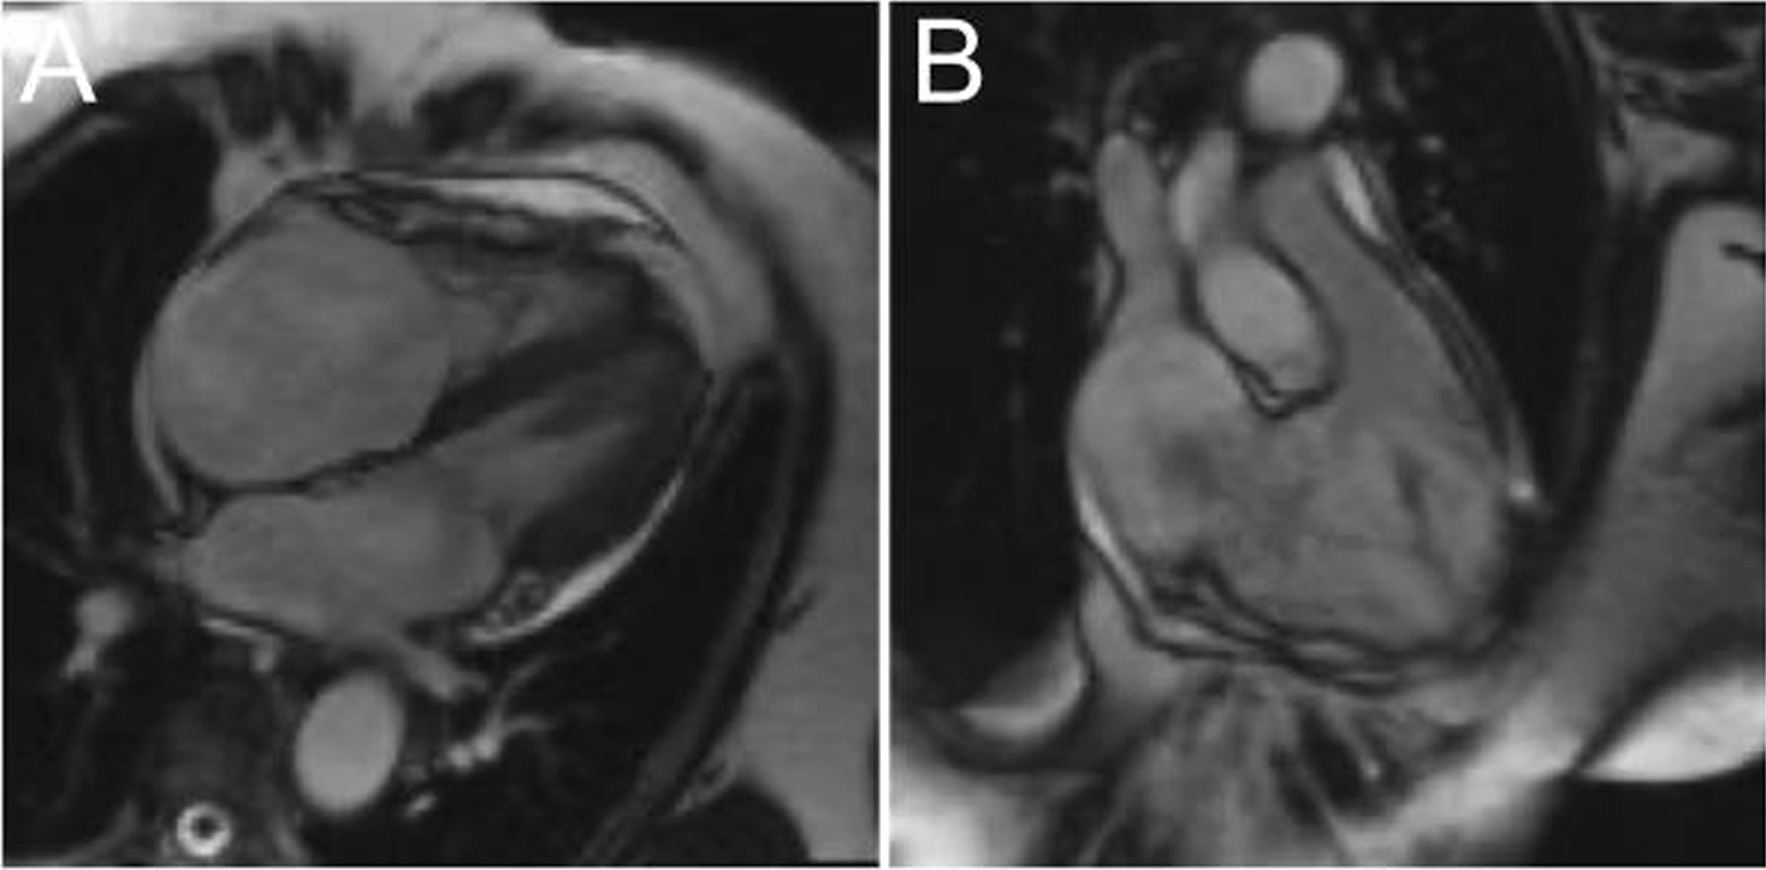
A MRI scan in four-chamber-view showing a highly dilated right atrium and a hypertrabeculized right ventricle. B MRI scan of the right ventricular outflow tract demonstrating the severe tricuspid regurgitation
